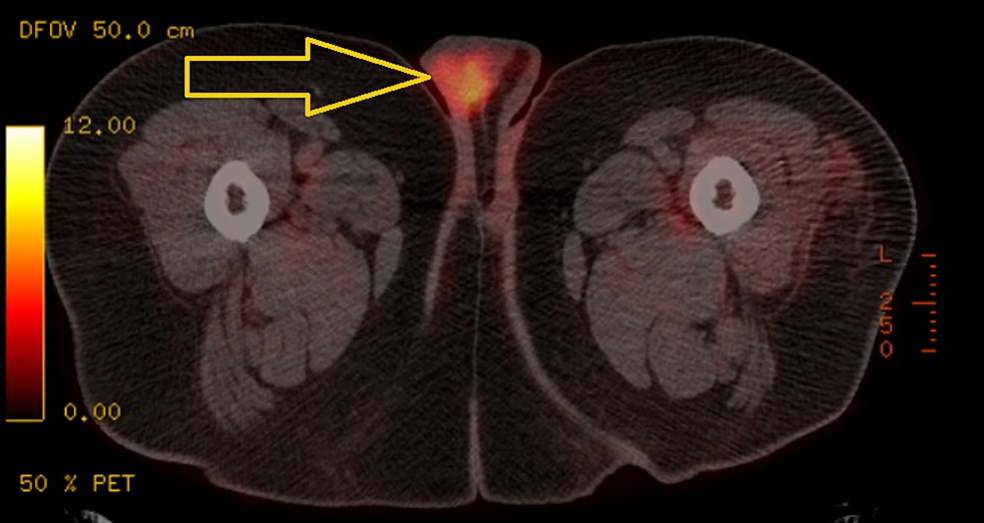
PET-CT, status post-bilateral orchiectomy, revealing an 8.0 SUV focus of hypermetabolic activity in the right hemiscrotum concerning for locally recurrent disease. PET-CT: positron emission tomography-computed tomography; SUV: standardized uptake value
